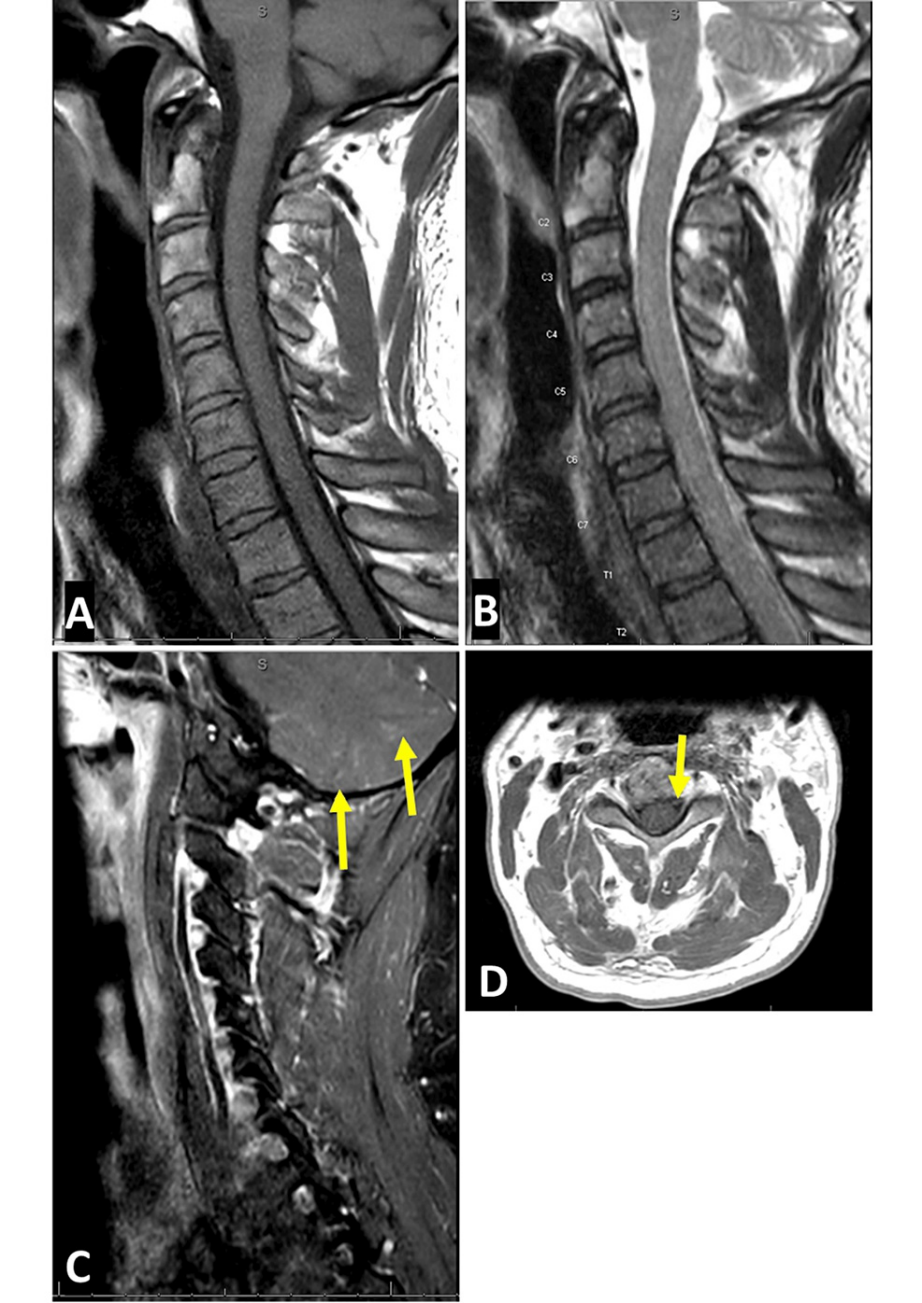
Sagittal T2 (A), T1 pre- (B) and post-contrast (C), as well as axial T1 post-contrast (D) MRI cervical spine from day 2 of hospitalization. The imaging shows subtle leptomeningeal enhancement surrounding the spinal cord and possibly some of the nerve roots. There is also linear and possibly nodular enhancement within the cerebellum predominantly in the cerebellar sulci suggestive of leptomeningeal enhancement (yellow arrow).
